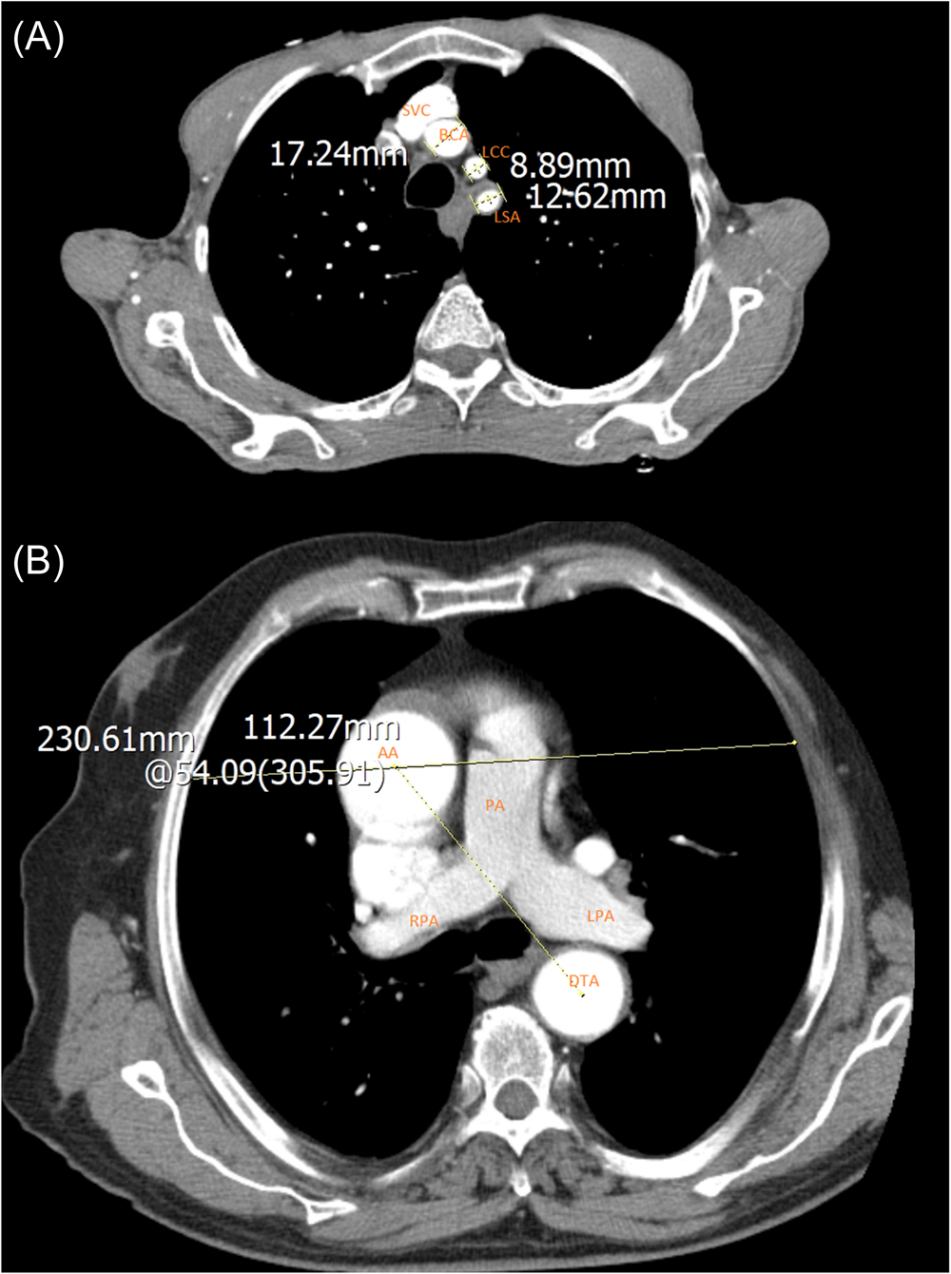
Computed tomography scan with Axial view, demonstrating applied measurements in our study. (A) The diameter of the brachiocephalic artery and the diameter of the left carotid artery at the proximal end; (B) The angle of curvature of the aortic arch relative to the coronal plane at the carina (demonstrating 62.38 degrees) (AA: Ascending aorta; DTA: descending thoracic aorta; BCA: brachiocephalic artery; LCC: left common carotid artery; LSA: left subclavian artery; LV: left ventricle; PA: pulmonary artery; LPA: left pulmonary artery; RPA: right pulmonary artery; CA: celiac artery; SMA: superior mesenteric artery).
